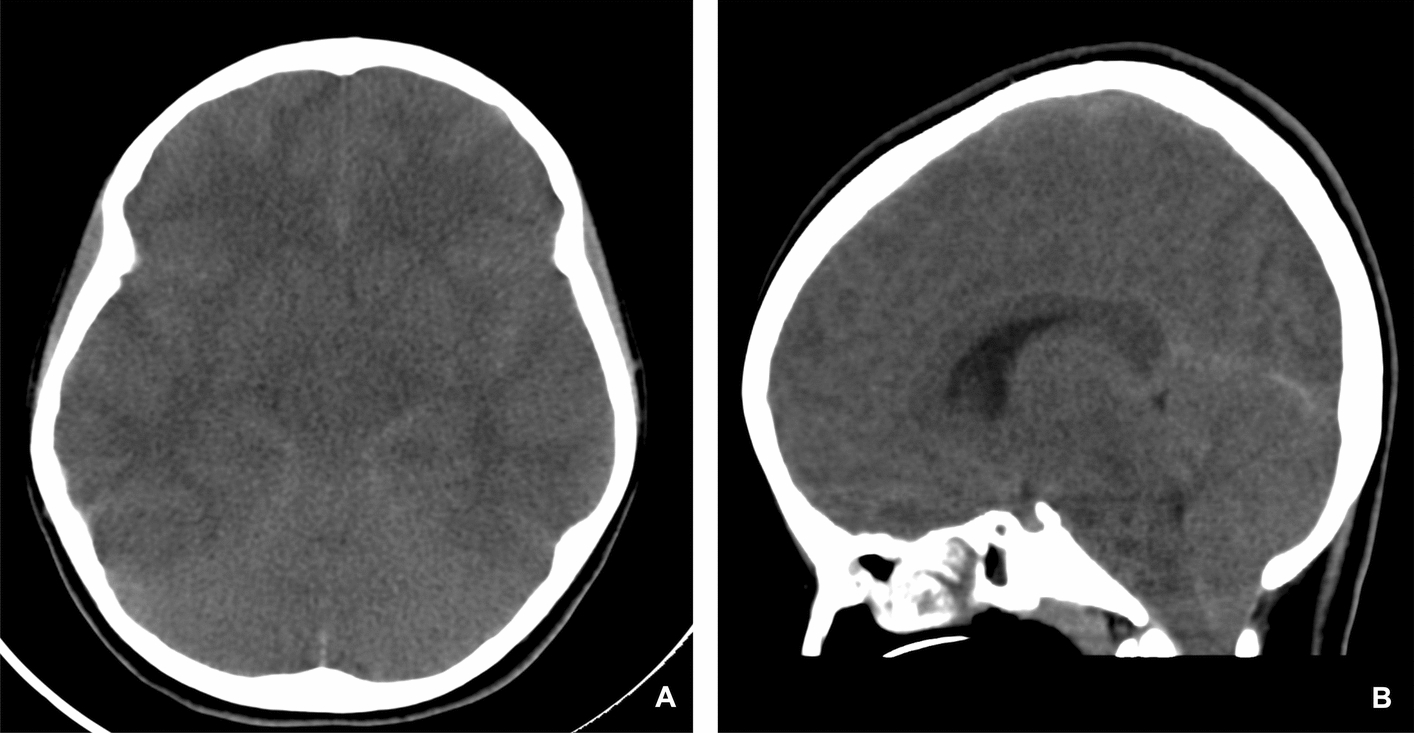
A Axial unenhanced computed tomography brain showing diffuse brain edema with effacement of the sulci and subarachnoid spaces. B Sagittal unenhanced computed tomography brain showing tonsillar herniation
